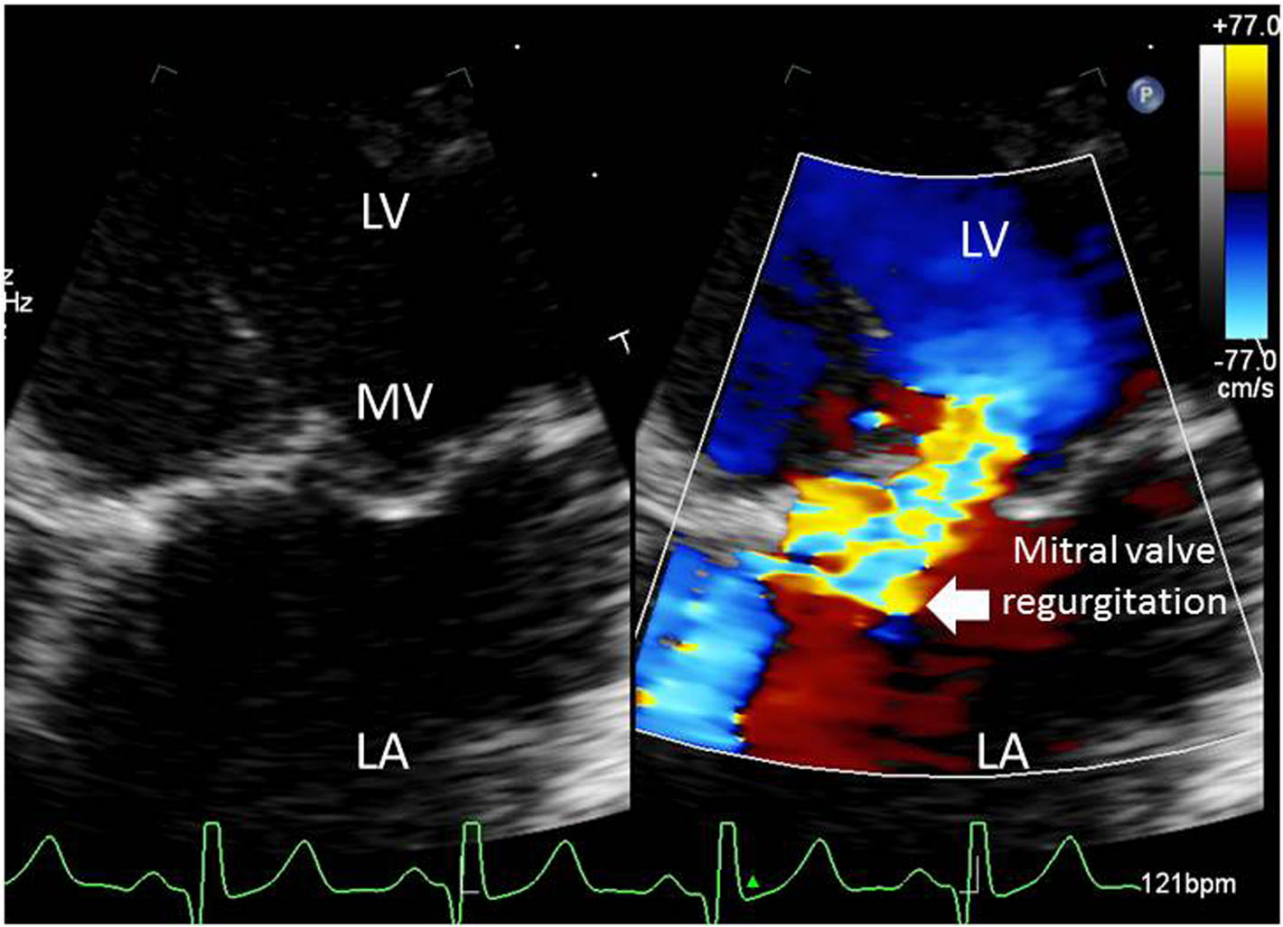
Doppler profile obtained at the mitral valve, showing severe regurgitation
